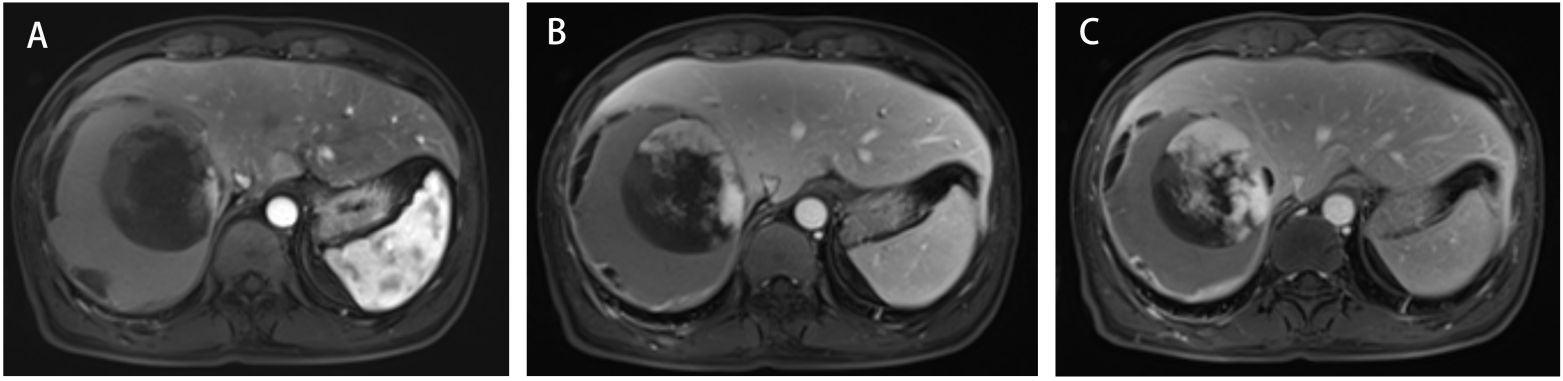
Three-phase enhanced MRI of the abdomen. (A) The arterial phase. (B) The portal venous phase. (C) The delayed phase.
